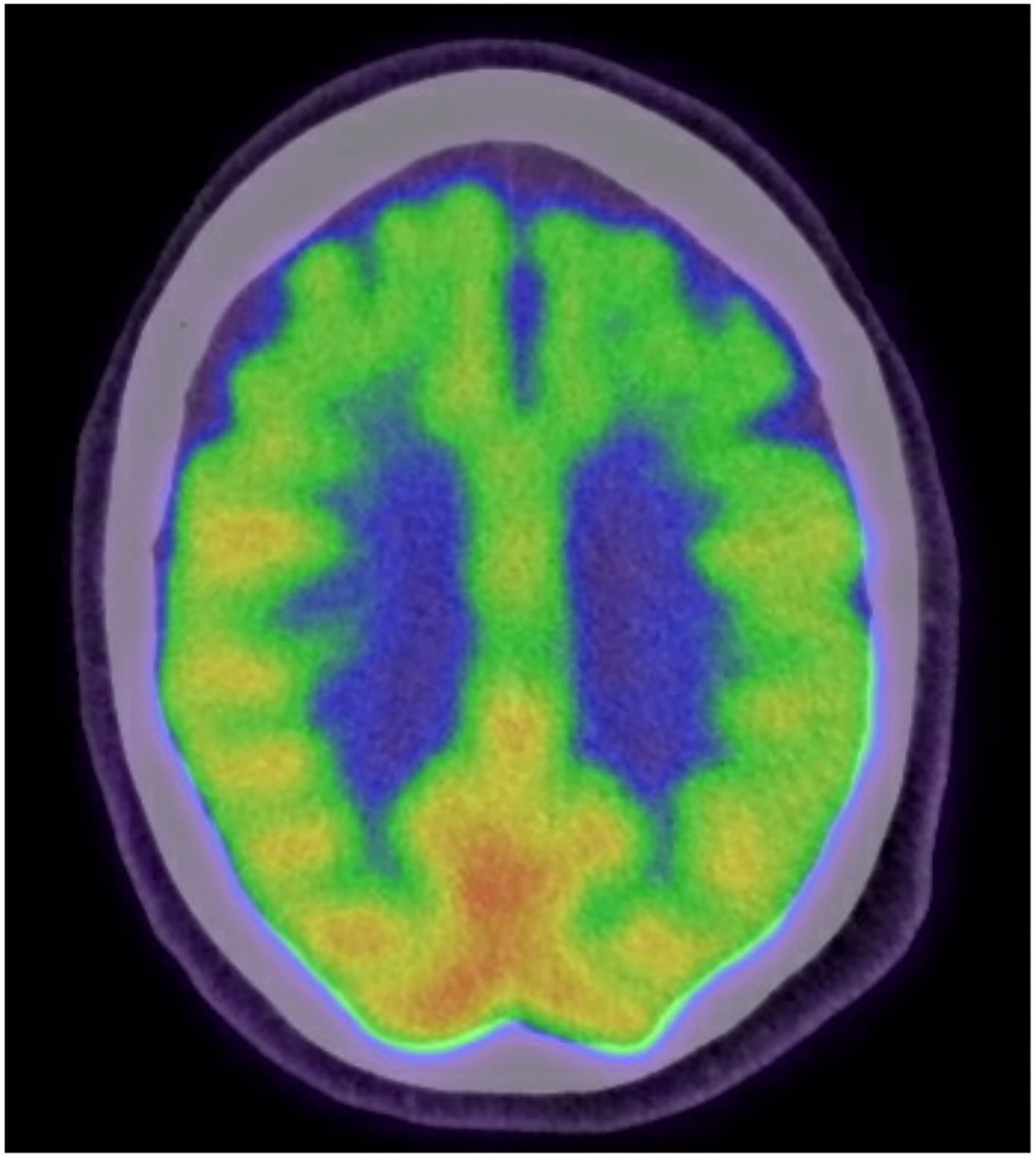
The patient's fluorodeoxyglucose (FDG)-positron emission tomography scan showing hypometabolic areas primarily in the left temporal lobe and biltateral frontal lobes.
